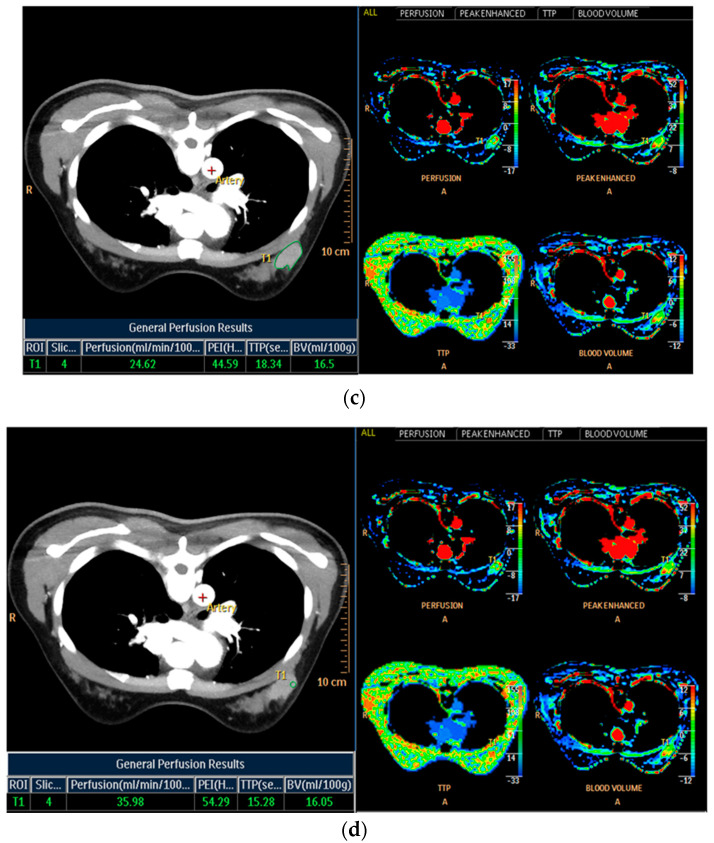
Histogram and perfusion analyses on breast CT in a 42-year-old woman with a 30 mm triple-negative invasive ductal carcinoma of the left breast (the same patient as Figure 2). (a,b) Histogram analysis on CT: Axial contrast-enhanced low-dose CT shows an oval shaped, irregular marginated, and heterogeneous enhancing mass. Histogram analysis on CT was performed using the same method on MRI. (c,d) Perfusion analysis on CT: Two ROIs (ROI1: entire tumor and ROI2: hot spot) were manually delineated and four perfusion parameters from each ROI were obtained, perfusion, peak enhancement intensity (PEI), time to peak (TTP), and blood volume (BV).
